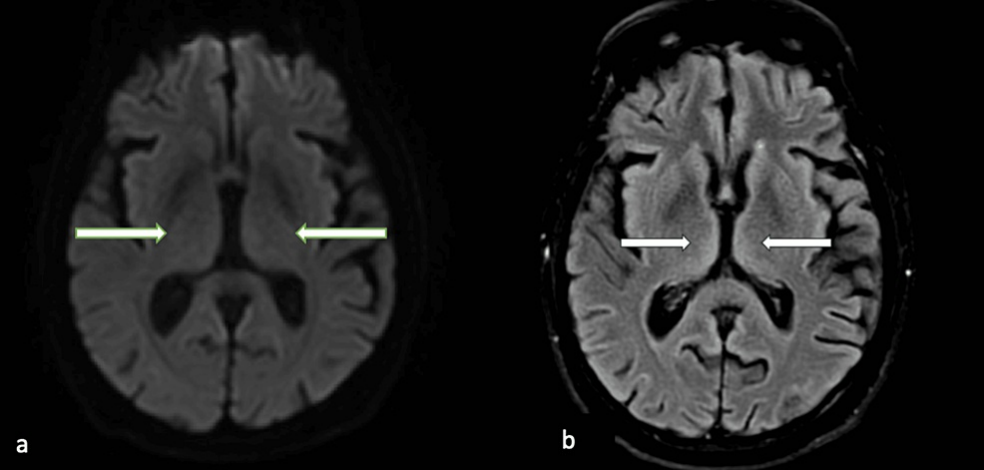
Repeat brain MRI nine days post-cardiac arrest a) Diffusion-weighted imaging brain MRI continued to show no diffusion restriction in bilateral thalami (see arrows). b) Significantly improved FLAIR signal in bilateral medial thalami (indicated by arrows). FLAIR: Fluid-attenuated inversion recovery
